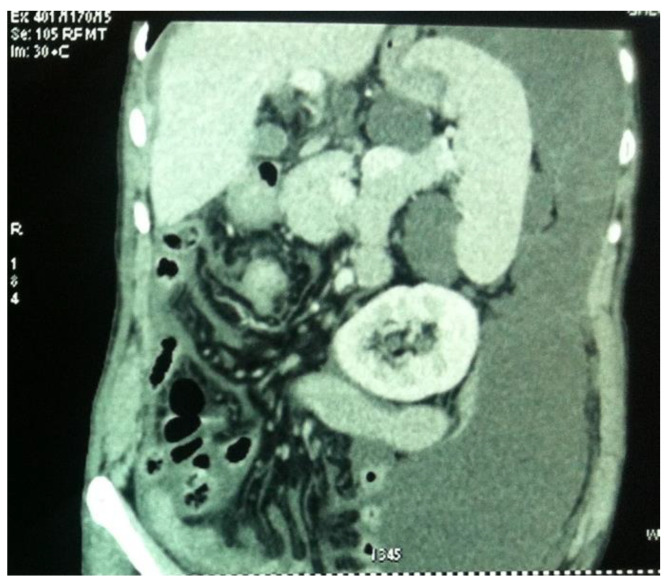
CT aspect of PP; evaluation of secondary peritoneal and intestinal metastases is affected by the presence of mucin in the left flank.
